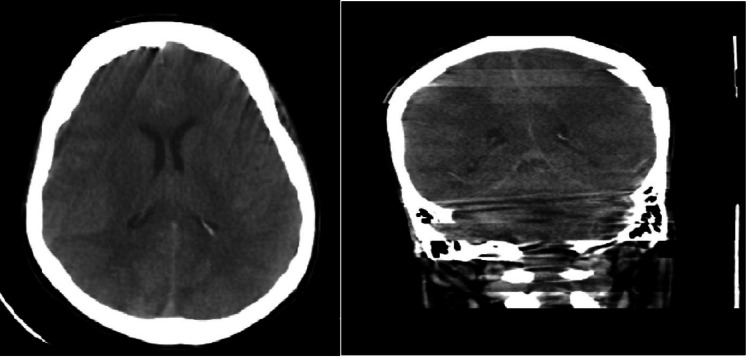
Computed tomography of the head showing no acute intracranial abnormalities
